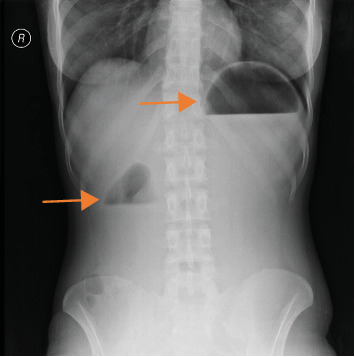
Plain abdominal X-ray showing dilated stomach and duodenal bulb with air–fluid level and paucity of gas in the remaining distal intestine.
